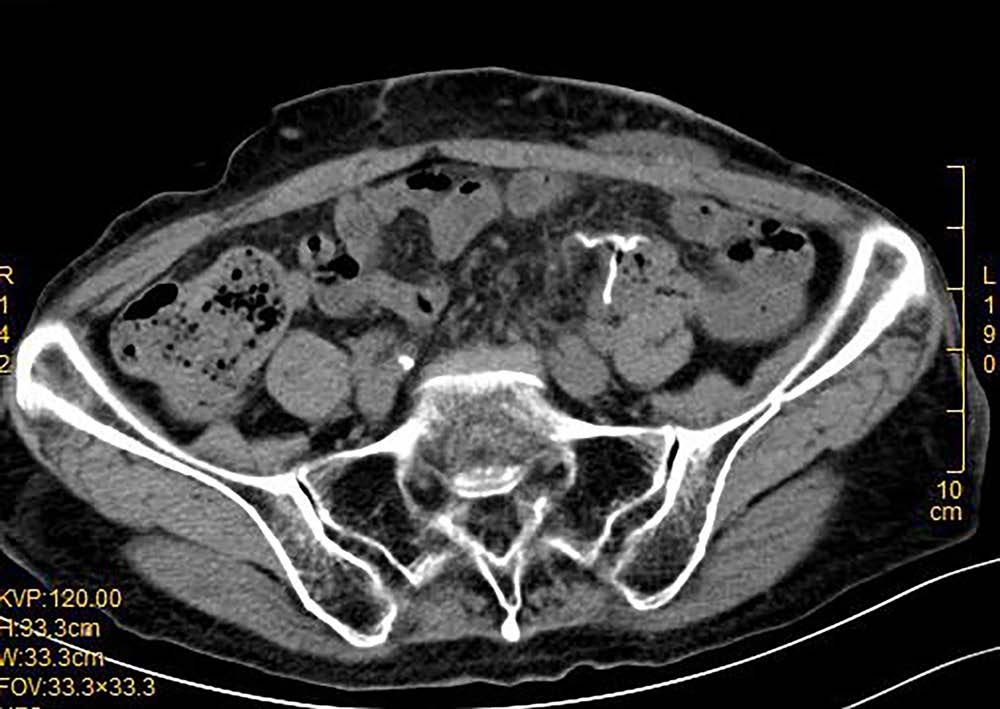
Two weeks postoperatively, follow-up CT scan demonstrated normal positioning of the anastomotic staples in the sigmoid colon, with well-defined surrounding fat planes. CT = computed tomography.
